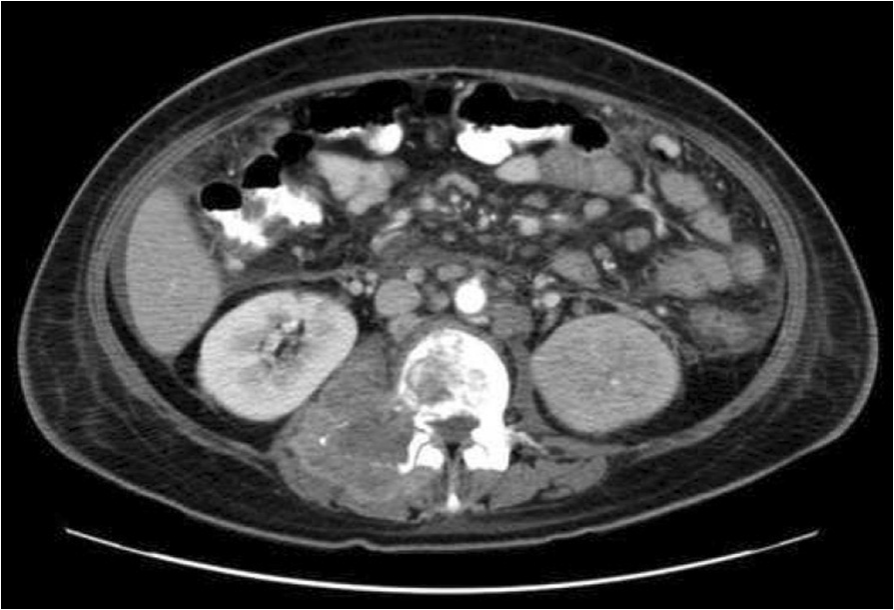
Computed tomography of the abdomen/pelvis with contrast showing a heterogenous 4-cm mass involving the body and pedicle of the L3 vertebra on the right; there is severe loss of vertebral body height and retropulsion of bony fragments into the spinal canal, creating severe stenosis.
